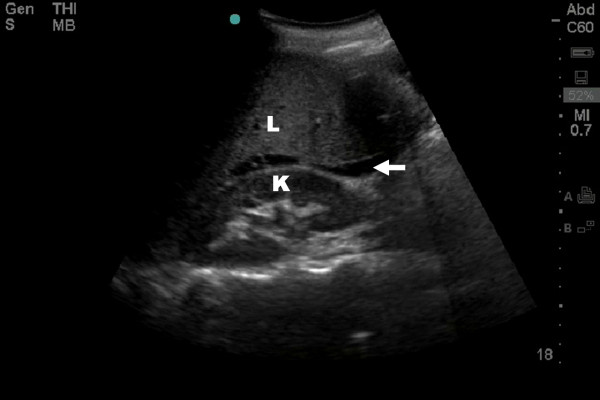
Resuscitative ultrasound image of hepatorenal fossae demonstrating free intra-peritoneal fluid seen as a hypoechoic stripe (arrow) between the liver (L) and kidney (K).
